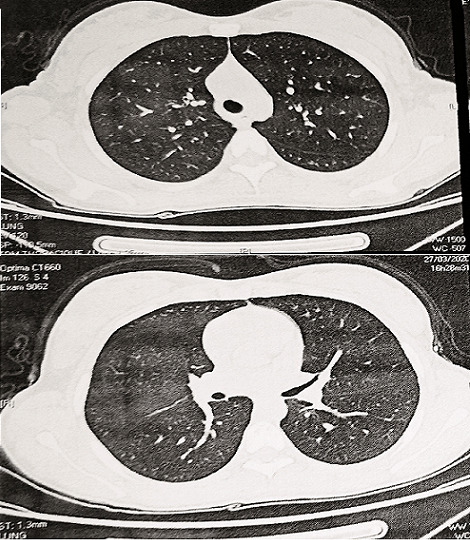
Chest CT scan in axial sections noting ground-glass opacities (patient 10)
